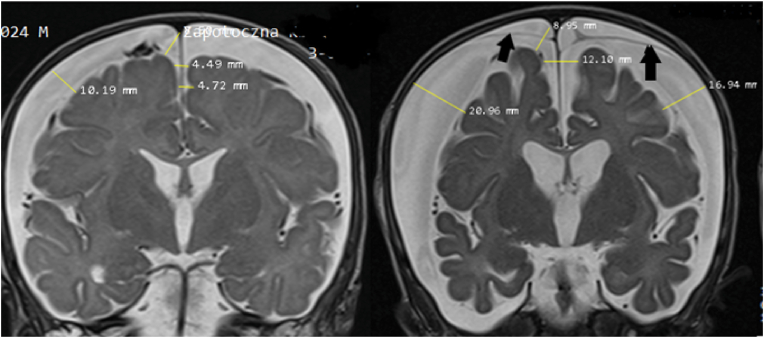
Case #2. Left: Coronal MRI scan at the level of Foramina Monroi 3 days after admission showing increased intracranial distances compatible with external hydrocephalus – complicated by a CSDH (Cranio-Cortical Width – CCW 10.1 mm, Sino-Cortical Width – SCW 8.5 mm, Inter-Hemispheric Width – IHW 4.7 mm) according to Lam et al. [24]. Note that the CSDH is not causing flattening of the cortical gyri or compression of the ventricles. Right: Coronal MRI scan at the same level five weeks later shows a dramatic increase of these intracranial distances, expansion of the fluid producing ventricles, and subdural neomembranes (black arrows) indicating that the subdural fluid collections are many weeks or even months old. The total width of the anterior horns of the lateral ventricles had increased from 26 mm to 34 mm.
